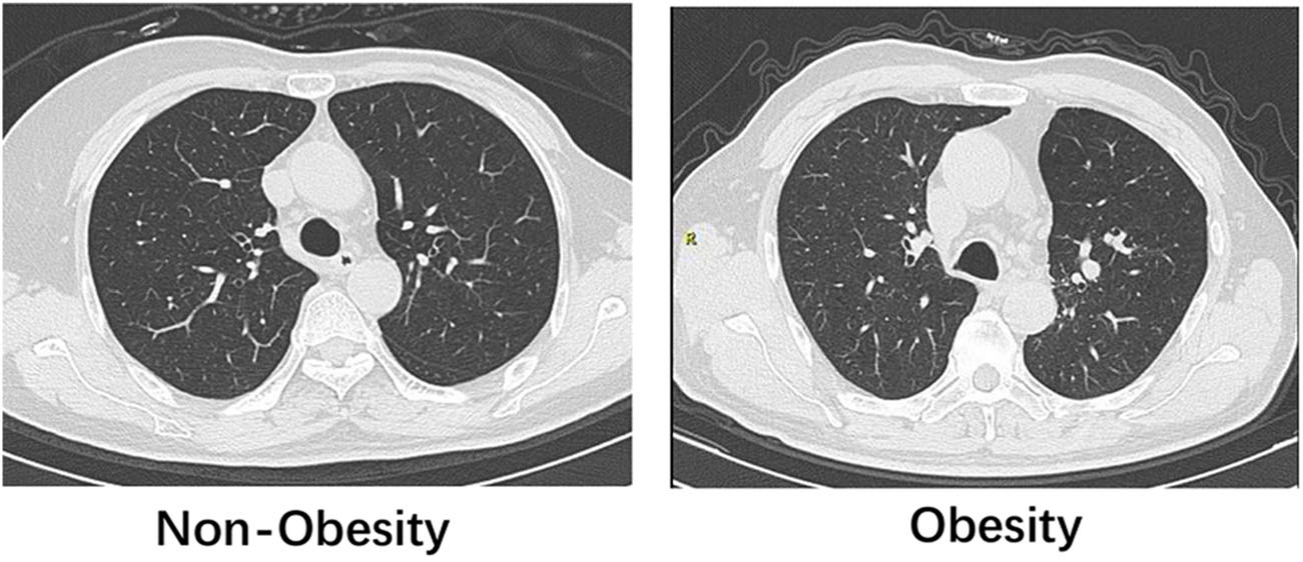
Chest computed tomography results of the nonobese and Obese patients
